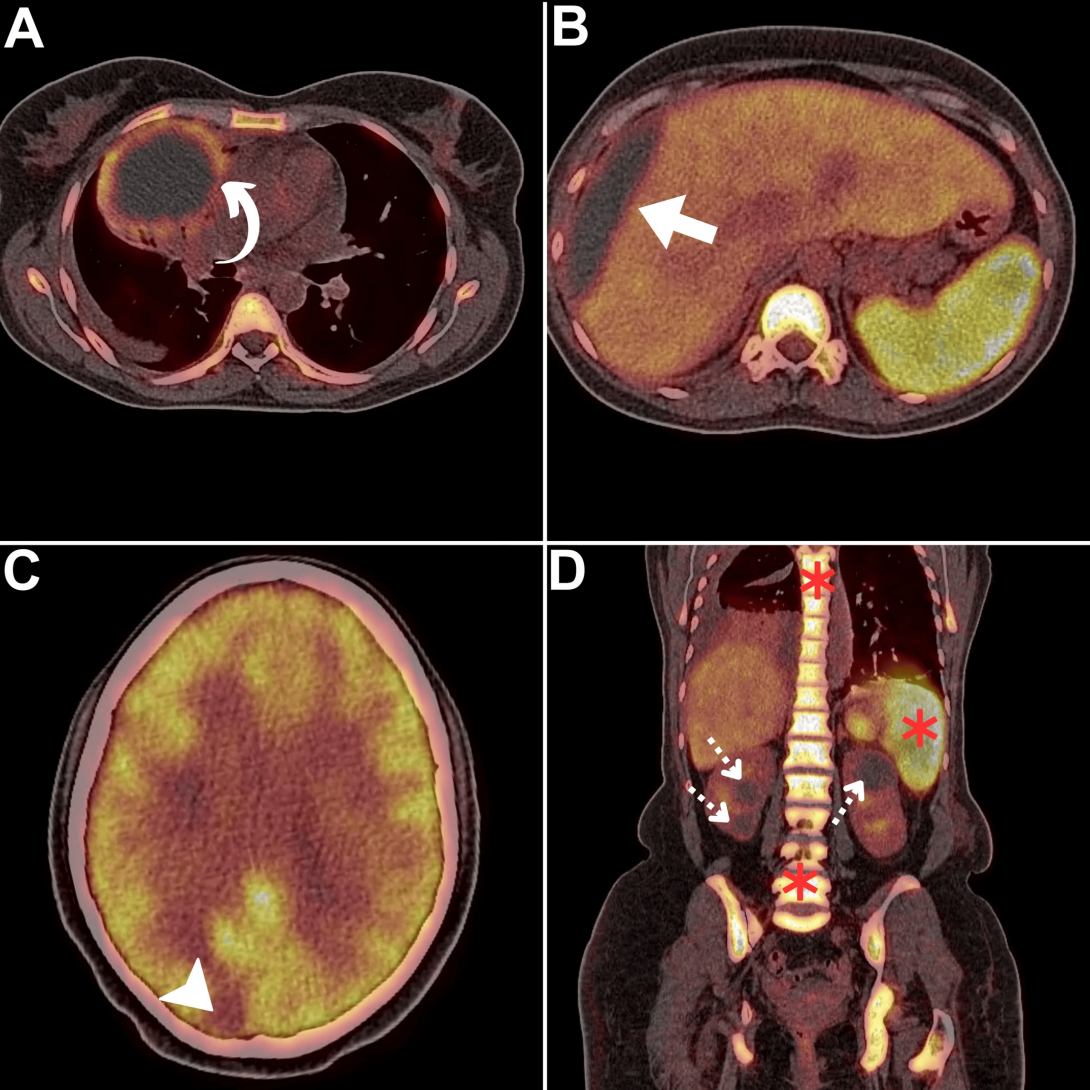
Baseline FDG PET/CT (A) Axial lung PET/CT revealed a single, large, peripherally hypermetabolic right upper lung lobe mass (curved arrow). (B, C) Axial PET/CT views demonstrated a large, hypometabolic occupying the lateral hepatic border (arrow) and brain (arrowhead). (D) Coronal PET/CT image exposed bilateral renal hypometabolic hypodensities (dotted arrows). Notably, intense bone marrow and splenic hypermetabolism was depicted (asterisks) correlating to ongoing anemia. FDG: fluorodeoxyglucose, PET/CT, positron emission tomography/computed tomography
